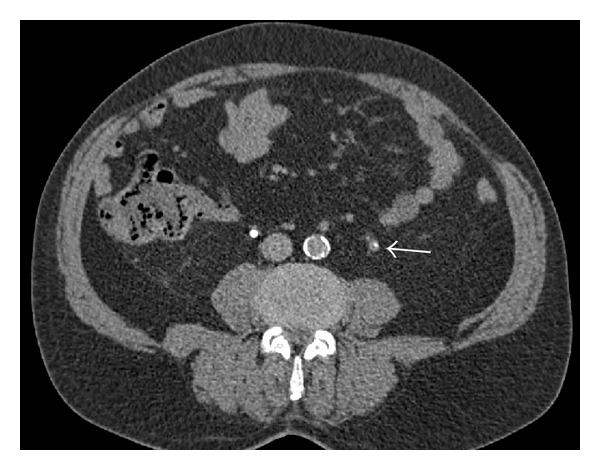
CT scan showing ureteral wall thickening with enhancement of contrast medium.
